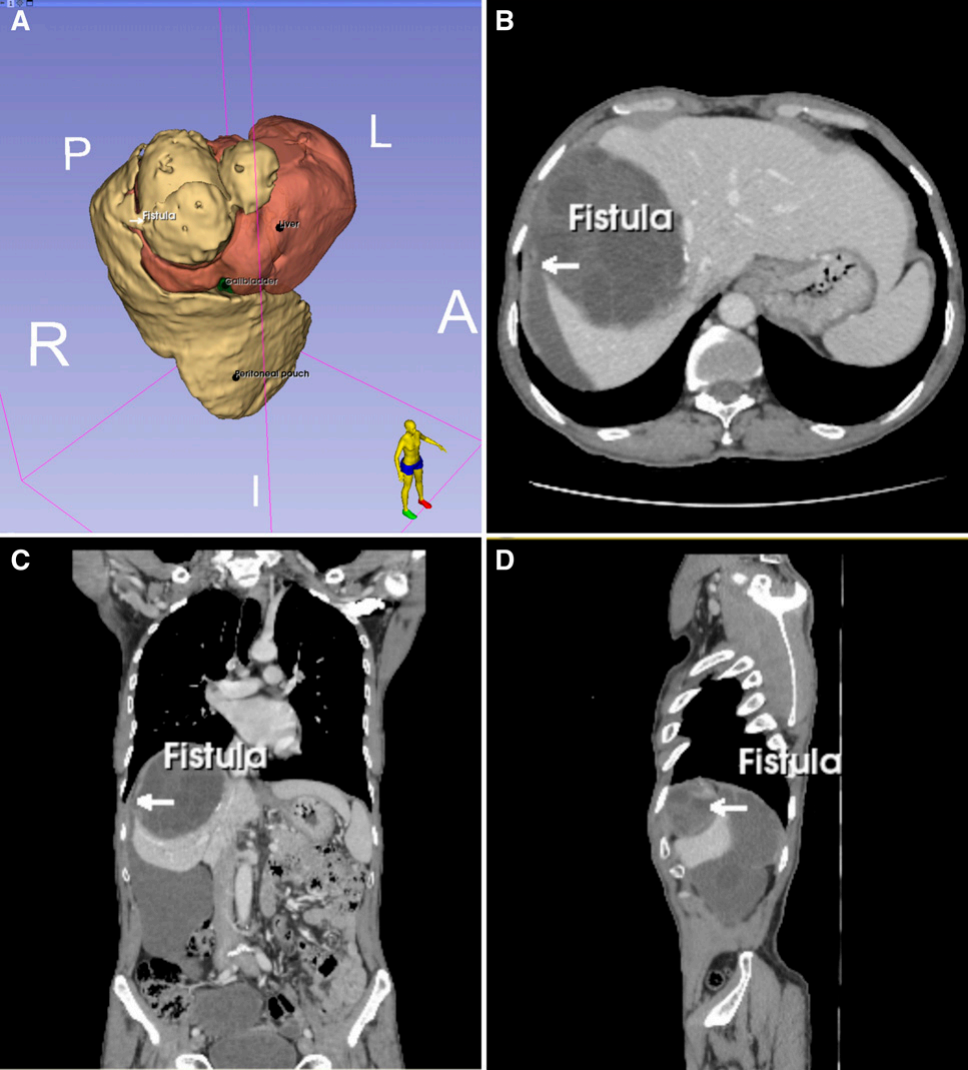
Computed tomography highlighting the fistulous path (white arrows). Three-dimensional reconstruction (A), axial view (B), coronal view (C), and sagittal view (D) showing the hepatic hydatid cyst and the pedunculated cyst in the right paracolic gutter, highlighting the fistulous path between them.
